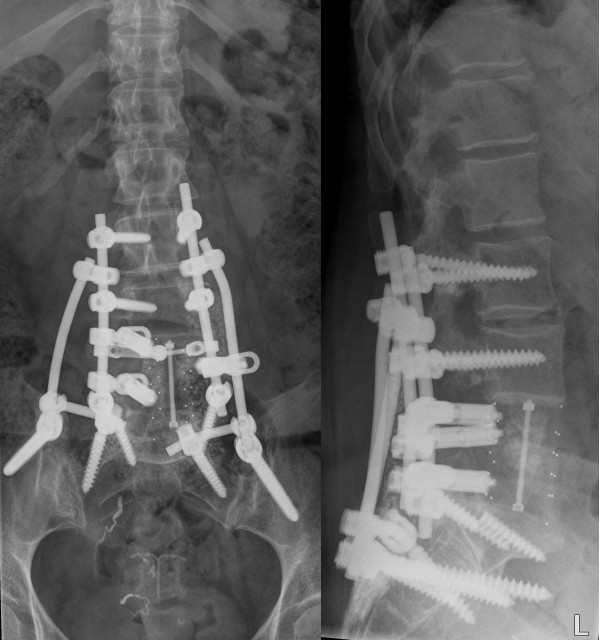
Case 2. A 42-year old patient with a pseudarthrosis of L5-S1 and failure of both S1 screws following en block spondylectomy for a chordoma of L5 who underwent extension of the posterior fusion with lumbosacral and spinopelvic fixation. Figure 3a: Postoperative anteroposterior radiograph. Figure 3b: Postoperative lateral radiograph
